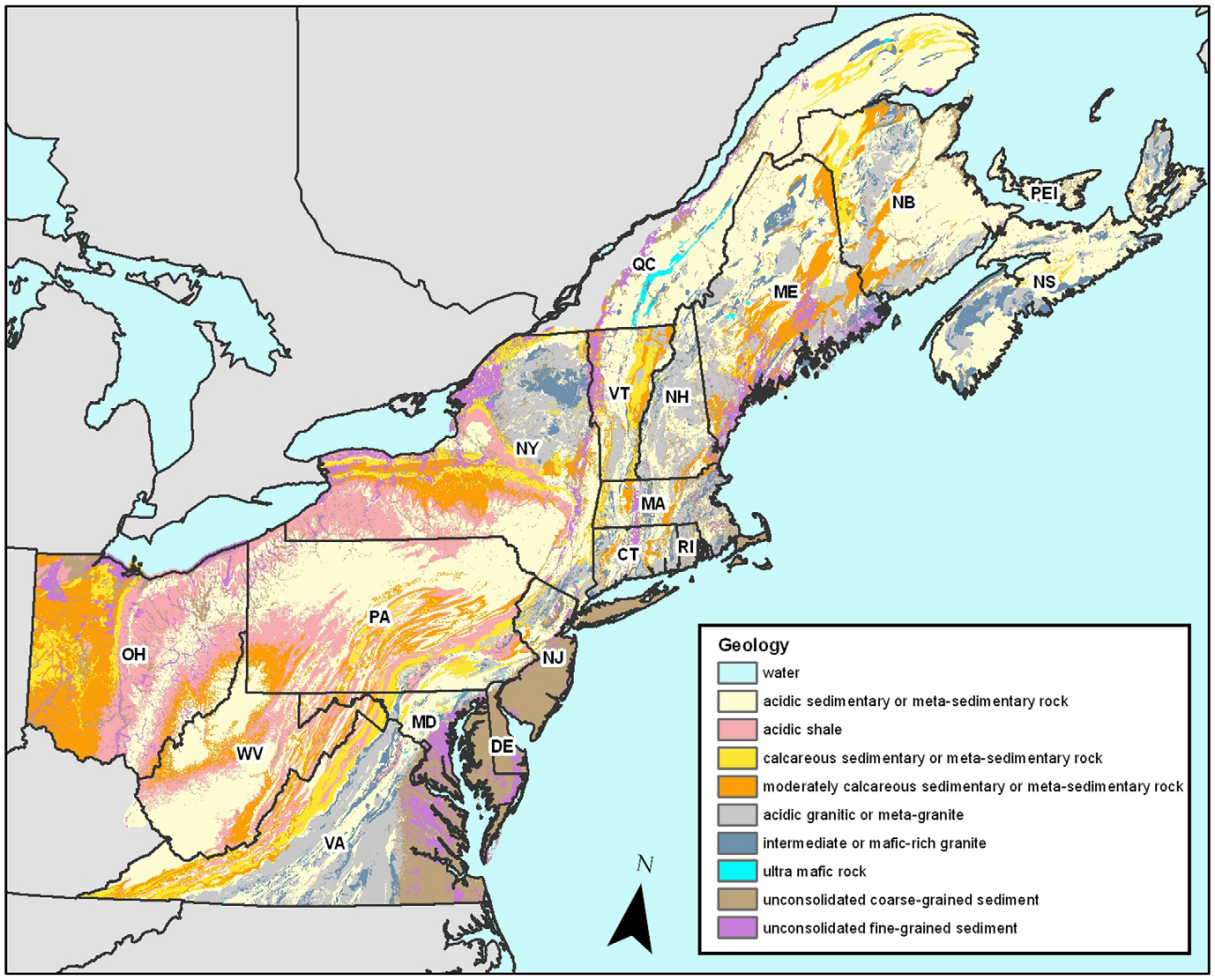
Map of the study region showing the geologic classes and state/province boundaries. Key to abbreviations: Maine (ME), New Hampshire (NH), Vermont (VT), New York (NY), Massachusetts (MA), Rhode Island (RI), Connecticut (CT), Pennsylvania (PA), Delaware (DE), New Jersey (NJ), Maryland (MD), Ohio (OH), West Virginia (WV), Virginia (VA), New Brunswick (NB), Nova Scotia (NS) and Prince Edward Island (PE).
